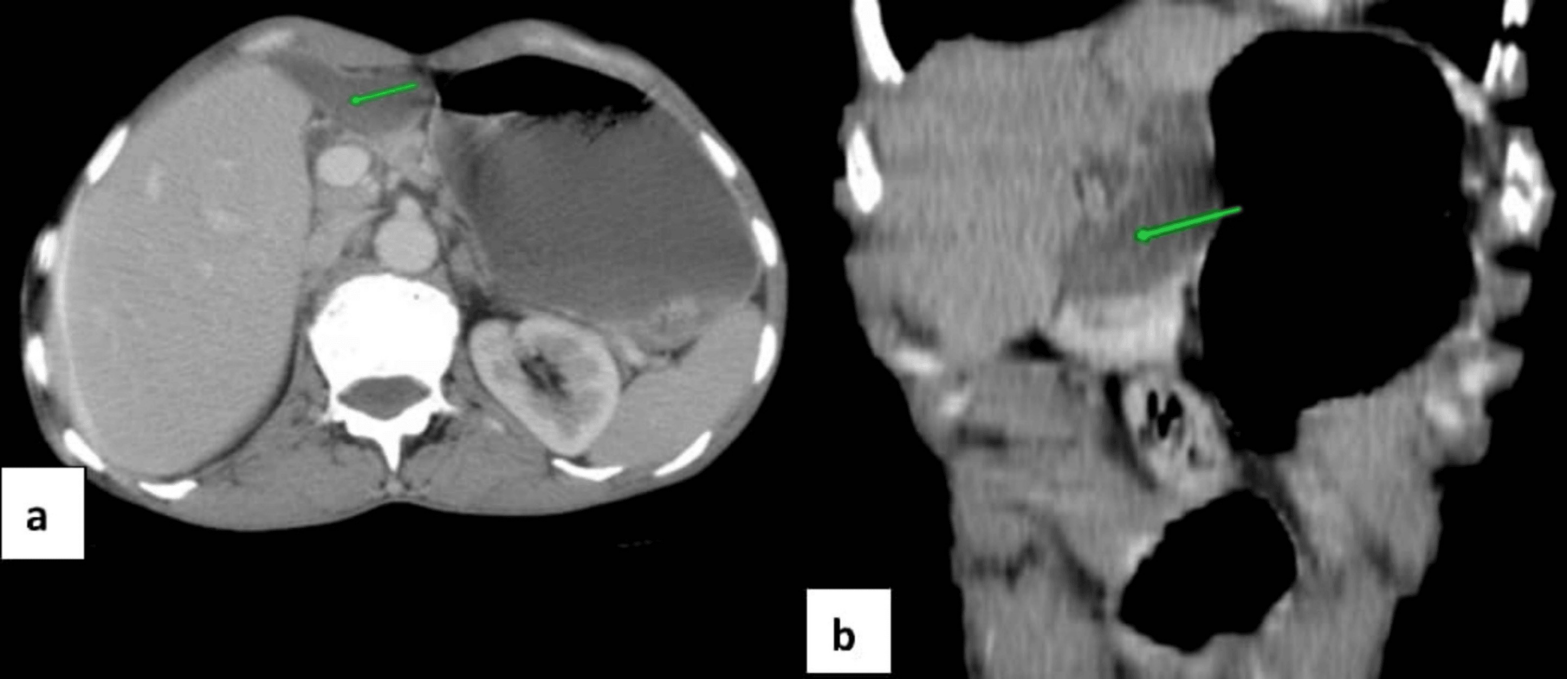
CT abdomen images demonstrating aberrant gallbladder position in the left subhepatic space Contrast-enhanced axial (a) and coronal (b) CT scans confirming the anomalous location of the gallbladder in the left subhepatic space (arrow indicates gallbladder)
